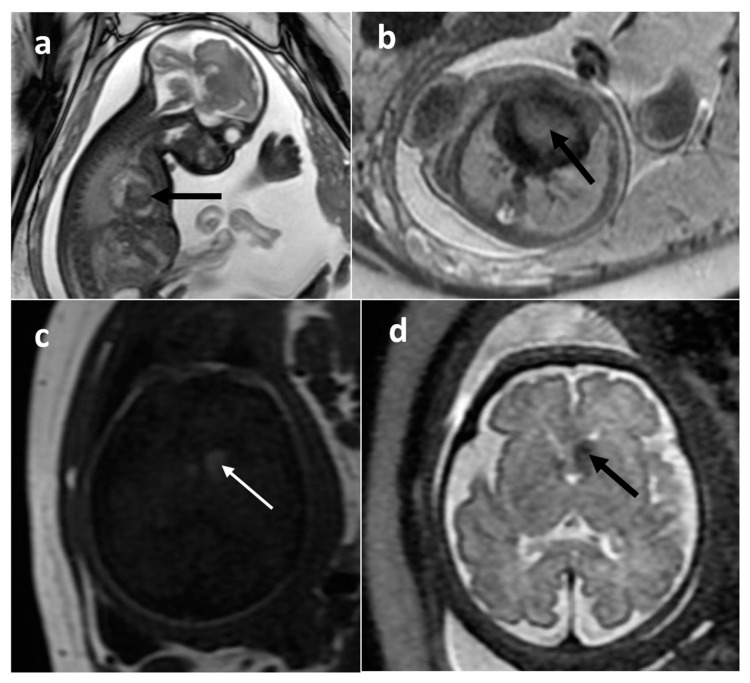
Tuberous sclerosis complex (TSC) on fetal MRI. Cardiac rhabdomyoma: (a) sagittal FIESTA; (b) axial SSFSE/T2. Subependymal giant cell astrocytoma (SEGA): (c) T1WI; (d) SSFSE/T2WI.
